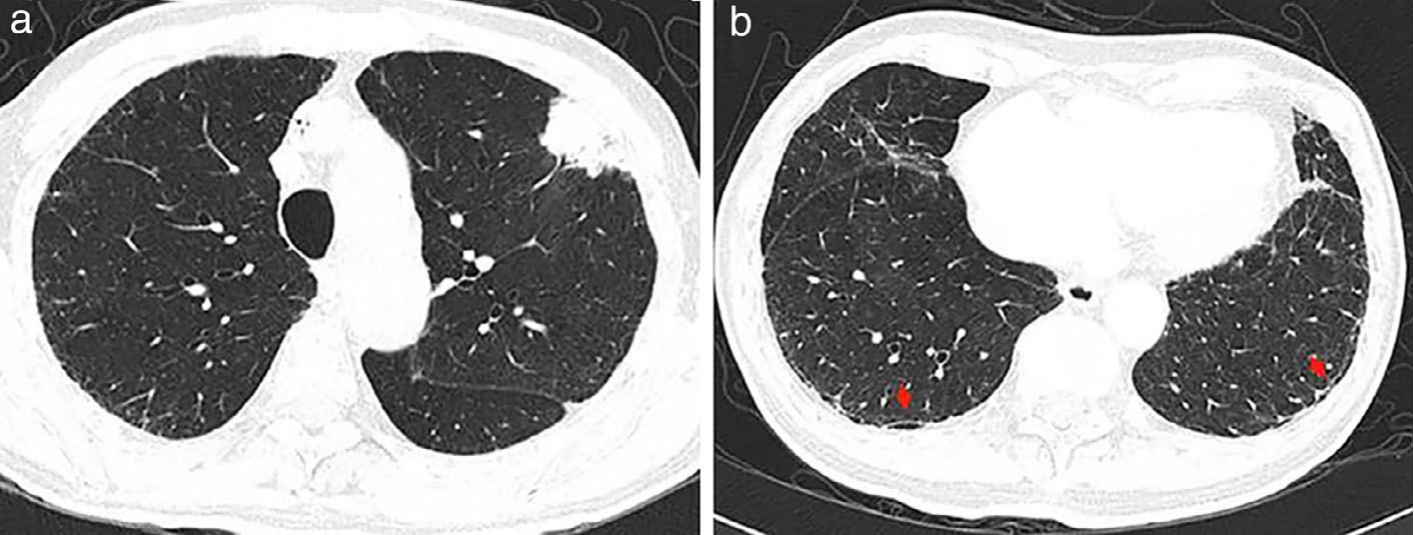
CT scan of the chest showed a pulmonary space‐occupying lesion in the left upper lobe highly suspicious of lung cancer. (a) A pulmonary space‐occupying lesion is visible in the left upper lobe. (b) Bilateral subpleural line of the lung indicated by red arrows.
